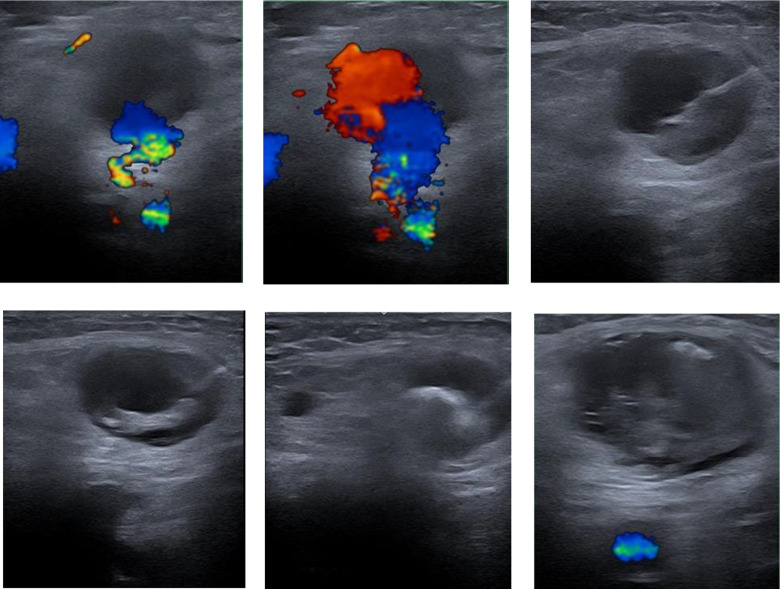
Pseudoaneurysm before UGFI; injection of the needle; instillation of UGFI; successfully thrombosis of the PSA after UGFI. UGFI indicates ultrasound-guided fibrin glue injection.
